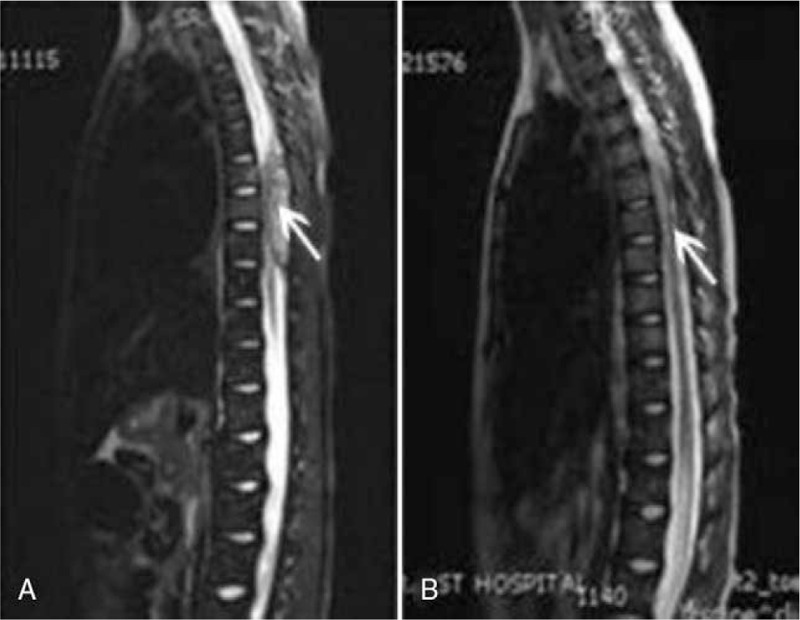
Spinal magnetic resonance imaging revealed a dorsal epidural mass from the level of T6 to T8 before and after radiotherapy.
